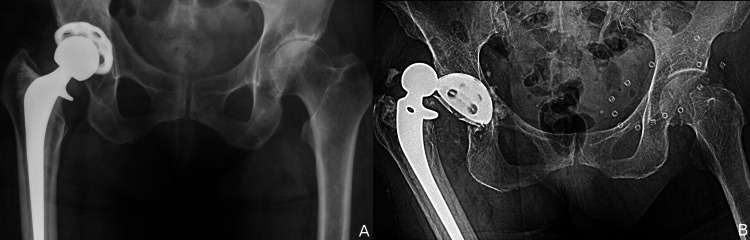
Older acetabular implants with locking mechanisms incompatible with a dual mobility liner required removal. (A) AP radiograph of a dislocating patient with a Duraloc Bantam cup (DePuy). (B) AP radiograph of a dislocating patient with an HG 2 cup (Zimmer).
